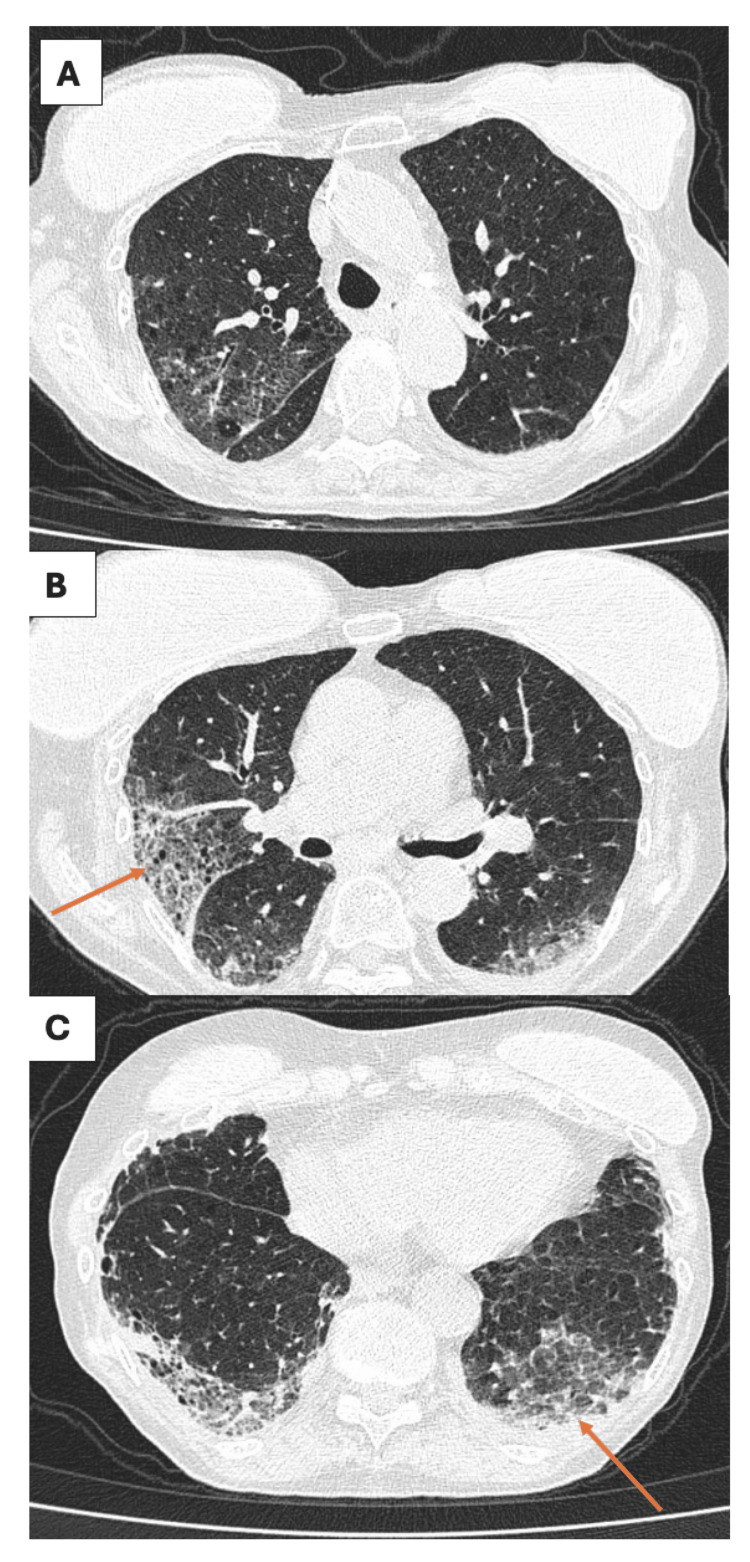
High-resolution follow-up CT imaging: Case 1 (B, C) Orange arrows depicting the progression in the lower lobe-predominant ground-glass opacities.
